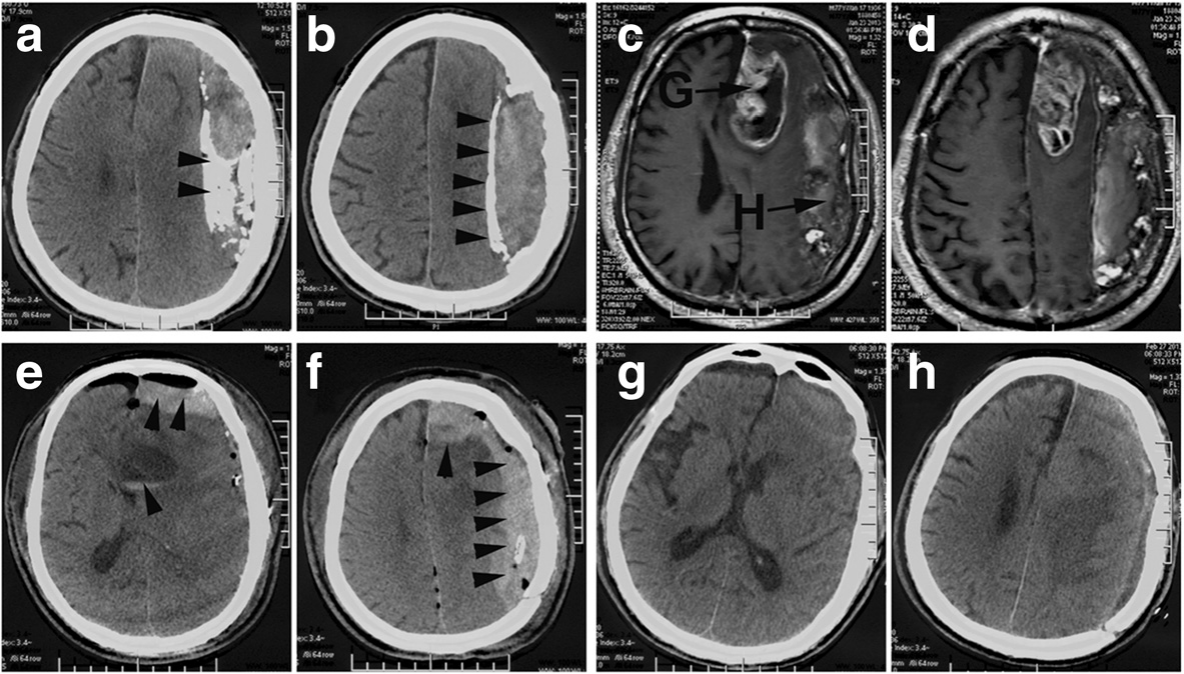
The preoperative and postoperative images of CT and MRI. (a,b) The CT images display the CSDH. The arrowheads point to the calcified membranes. (c, d) The MRI images show the GBM (G) and the CSDH (H). (e, f) The CT images indicate the *in-situ* hemorrhages after removal of the GBM and CSDH. (g, h)The postoperative CT images of the secondary craniotomy.
